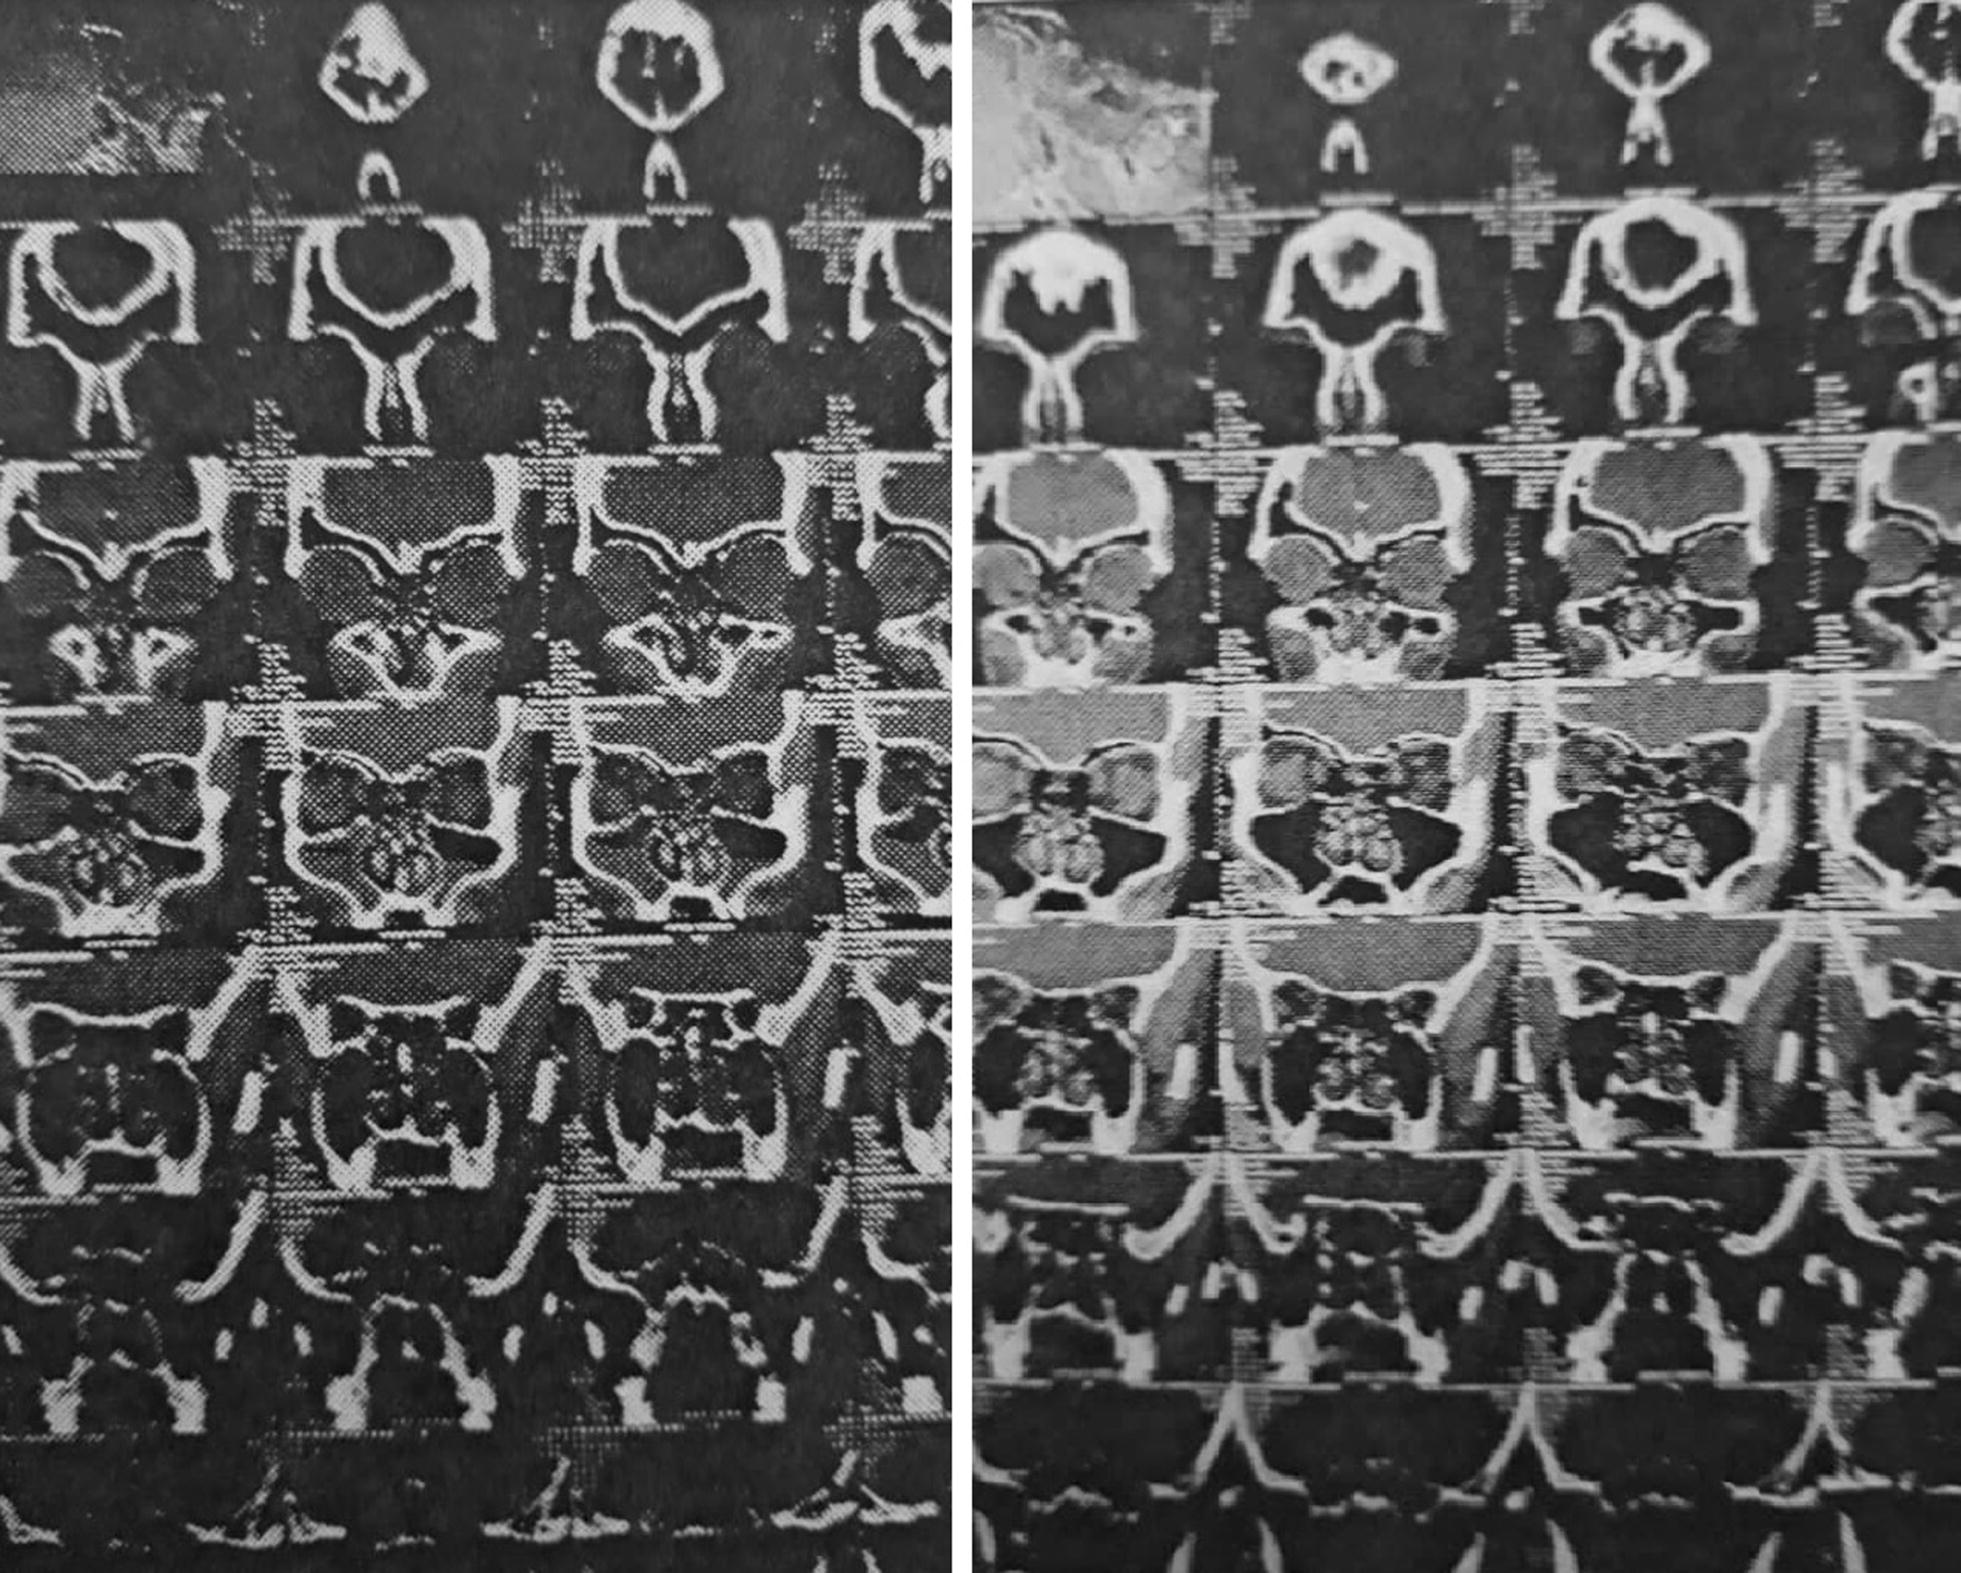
Pre-treatment (left) and post-treatment (right) findings by CT scan
